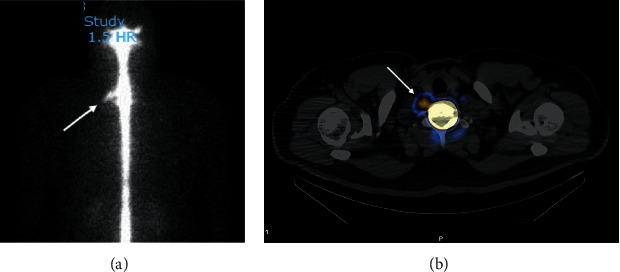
(a) Radionuclide (Tc-99 DTPA) cisternogram at 3-hour interval showed Tc-99 DTPA tracking out at the right of the upper cervical/thoracic vertebrae (white arrow). (b) SPECT at 2-hour time interval, axial view, showed focal extrathecal tracer accumulating at the right anterolateral aspect of the C7/T1 level (white arrow).
